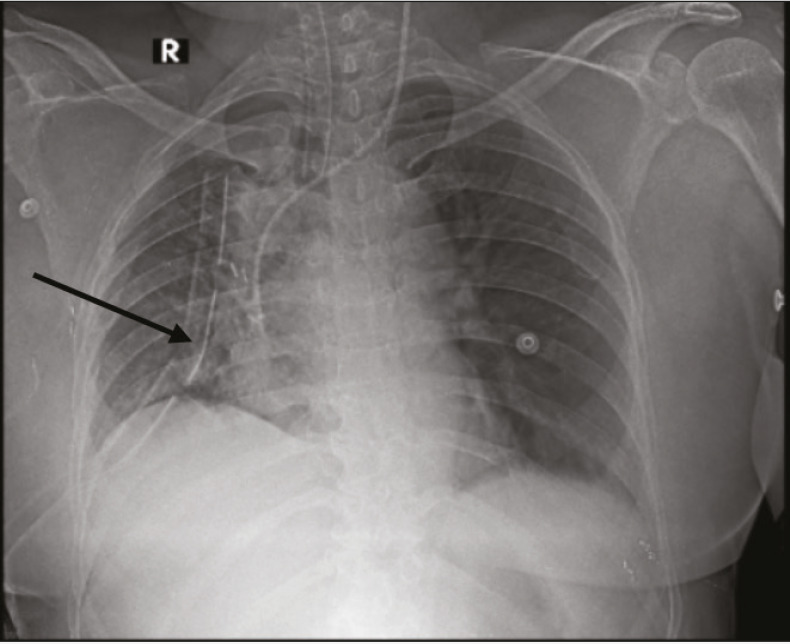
Postoperative chest X-ray after re-exploration, showing re-inflation of the right lower lobe. R: right.
